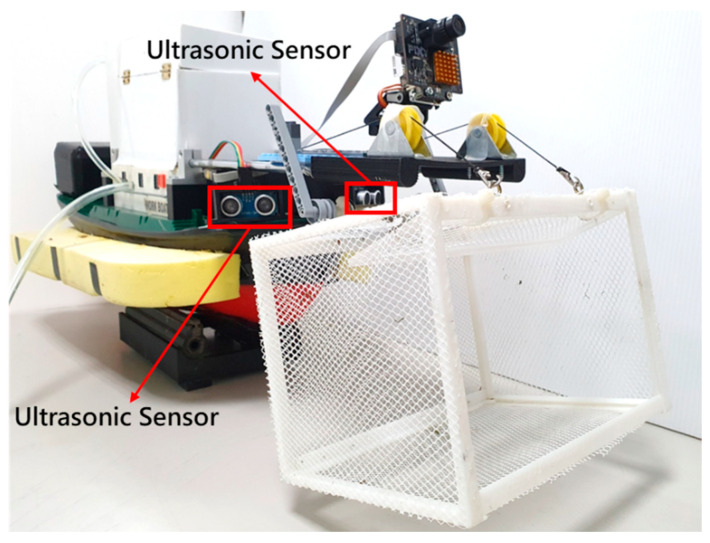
Ultrasonic sensors mounted on the MF-USV.
